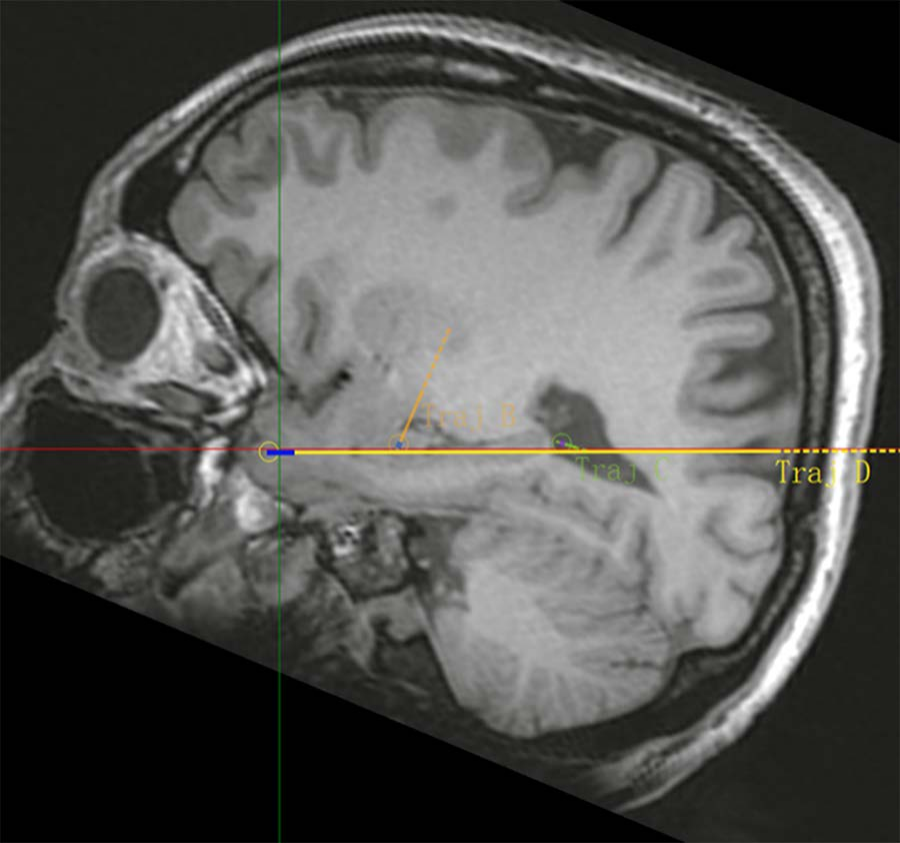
MRI T1 sequence sagittal view.
